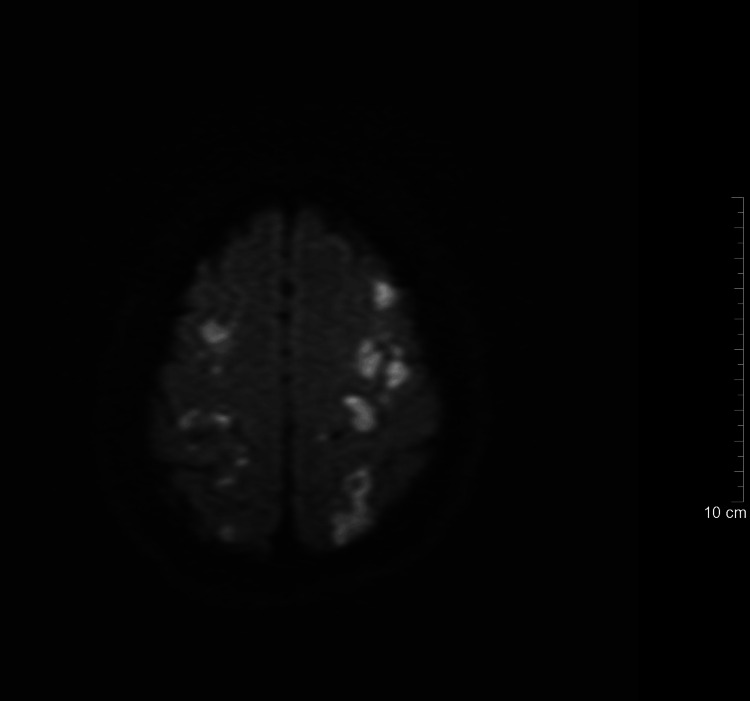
Pretreatmen diffusion-weighted MRI demonstrating multiple hyperintense lesions in the bilateral frontal and parietal lobes, consistent with septic emboli with cerebral microabscess formation. Axial diffusion-weighted MRI obtained at admission demonstrates multiple hyperintense lesions within the bilateral frontal and parietal subcortical white matter, consistent with septic emboli resulting from hematogenous spread of Staphylococcus aureus originating from a tunneled hemodialysis catheter.
